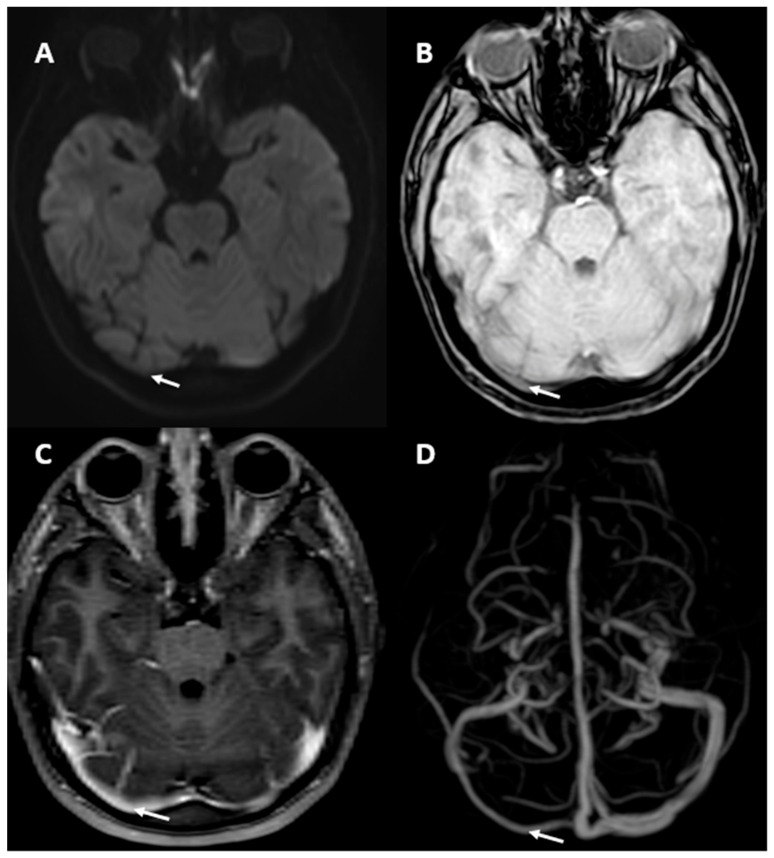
MR imaging findings of right transverse sinus hypoplasia in a representative case, shown in direct comparison with the thrombosis-specific signals demonstrated in Figure 2. The right transverse sinus is indicated by thick white arrows in all images. (A) DWI demonstrates no intraluminal restricted diffusion. (B) SWI shows no susceptibility-related blooming artifact. (C) CE-3D T1WI reveals no intraluminal filling defect. (D) MIP-CE-MRV confirms absence of filling defect with preserved sinus patency. The absence of thrombosis-specific MRI findings across all sequences is consistent with congenital hypoplasia rather than thrombotic occlusion, in contrast to the positive DWI (Figure 2E) and SWI (Figure 2F) findings observed in the thrombosis case.
